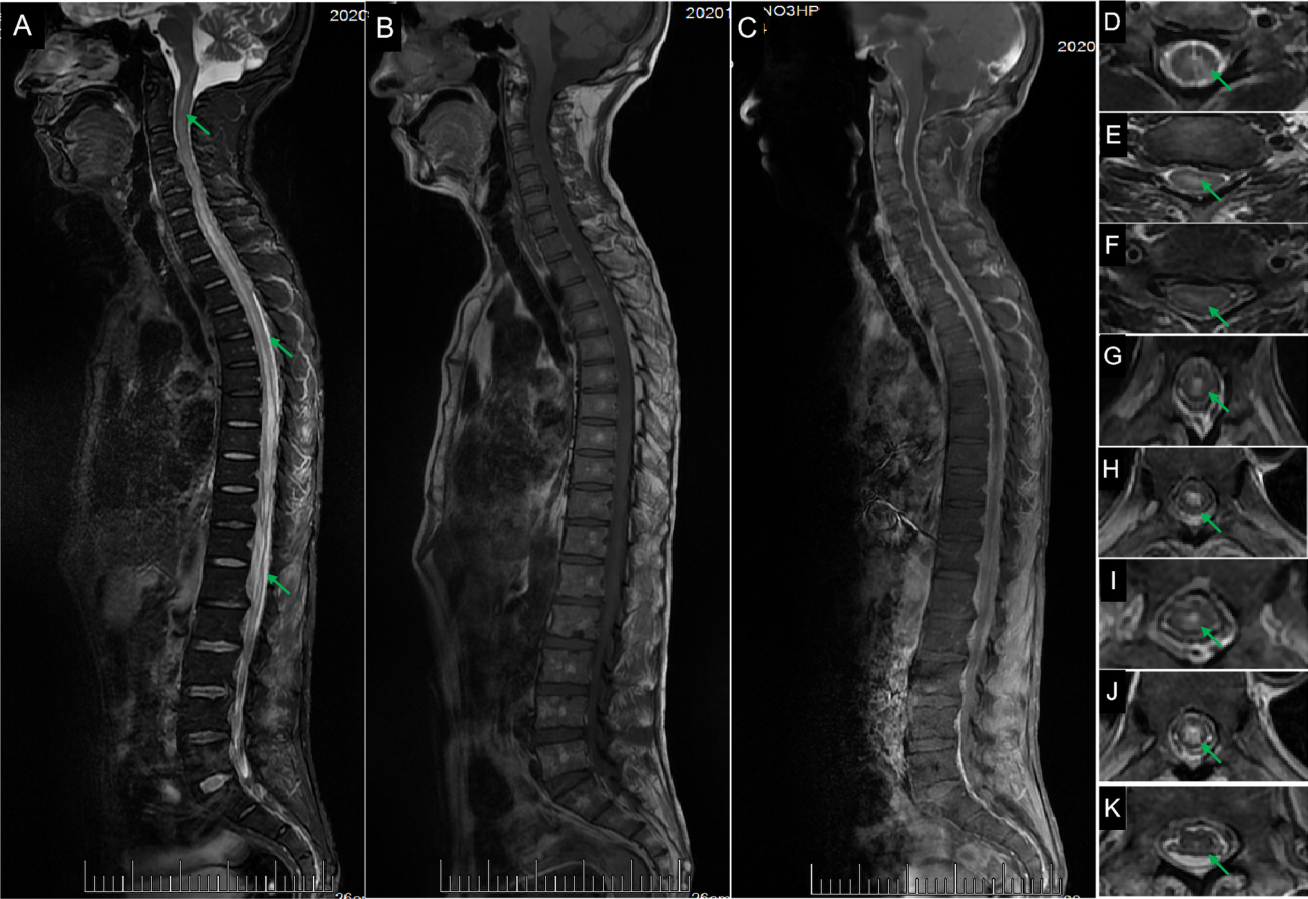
Spinal cord magnetic resonance imaging. Spinal cord magnetic resonance imaging shows hyperintensities (A, arrows) within the cervical, thoracic, and lumbar spinal cord on T2-weighted imaging (WI), and the corresponding lesions are hypointense (B) on T1-WI. Lesions are not enhanced on gadolinium-enhanced imaging (C). Axial T2-WI imaging shows intramedullary hyperintensity and edema, involving mainly the central part and sparing the peripheral spinal cord at different levels (C–J)
